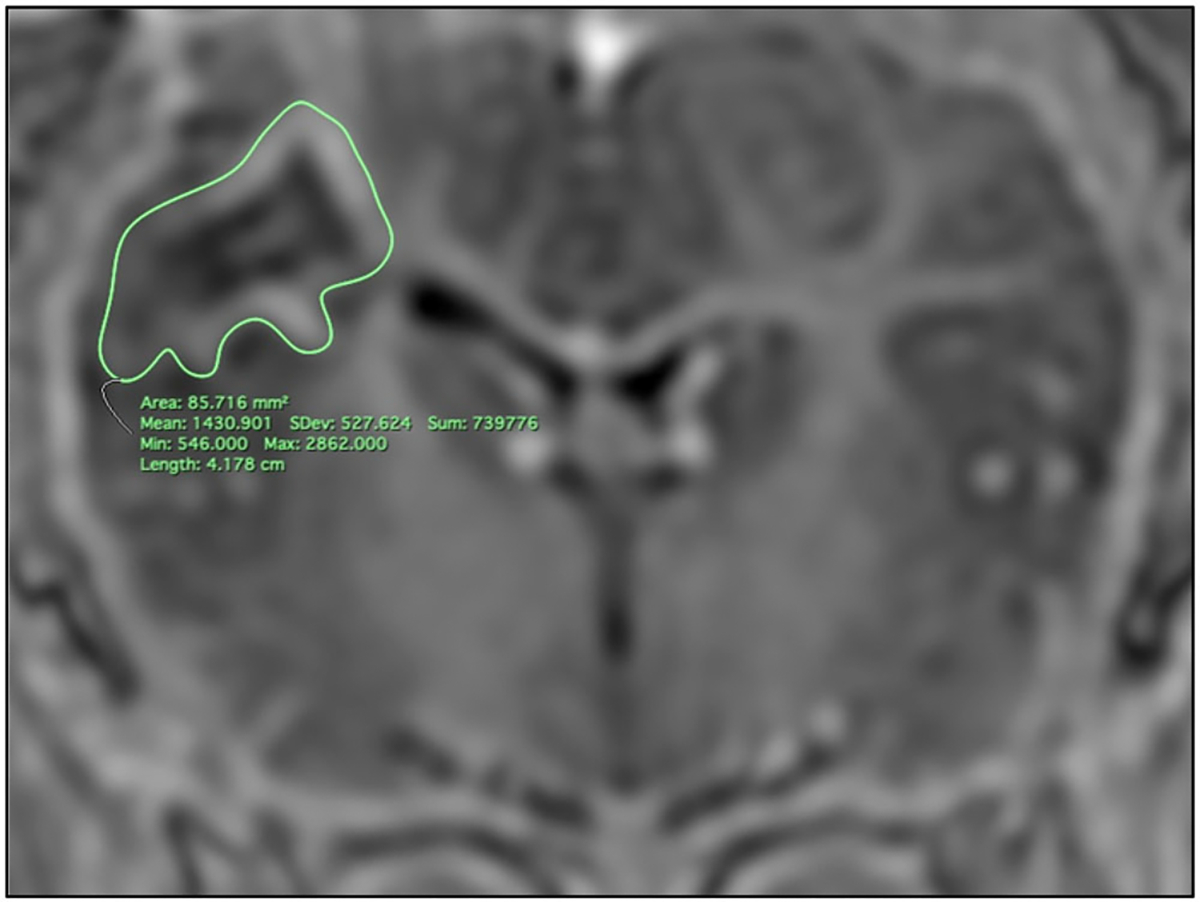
Coronal T1-weighted MRI with contrast 2 weeks post-ablation demonstrating the method for measuring the ablative lesion created by the 180° probe (4 W, 180 s) with the probe facing anteriorly. The lesion is seen here circled in green and appears as an area of hypo-intensity with a rim of surrounding contrast enhancement.
